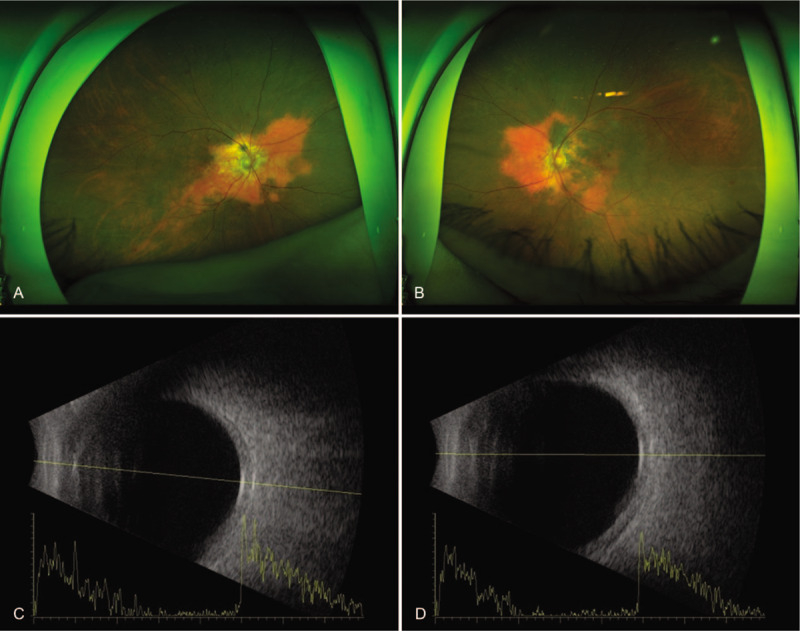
Ultra-widefield fundus photographs of the right (A) and left (B) eyes reveal well-demarcated, red-yellow colored lesion at the peripapillary area involving decalcification (yellow–white colored area). B-scan ultrasonography of the right (C) and left (D) eyes demonstrate a highly reflective choroidal mass, indicating bilateral choroidal osteomas.
